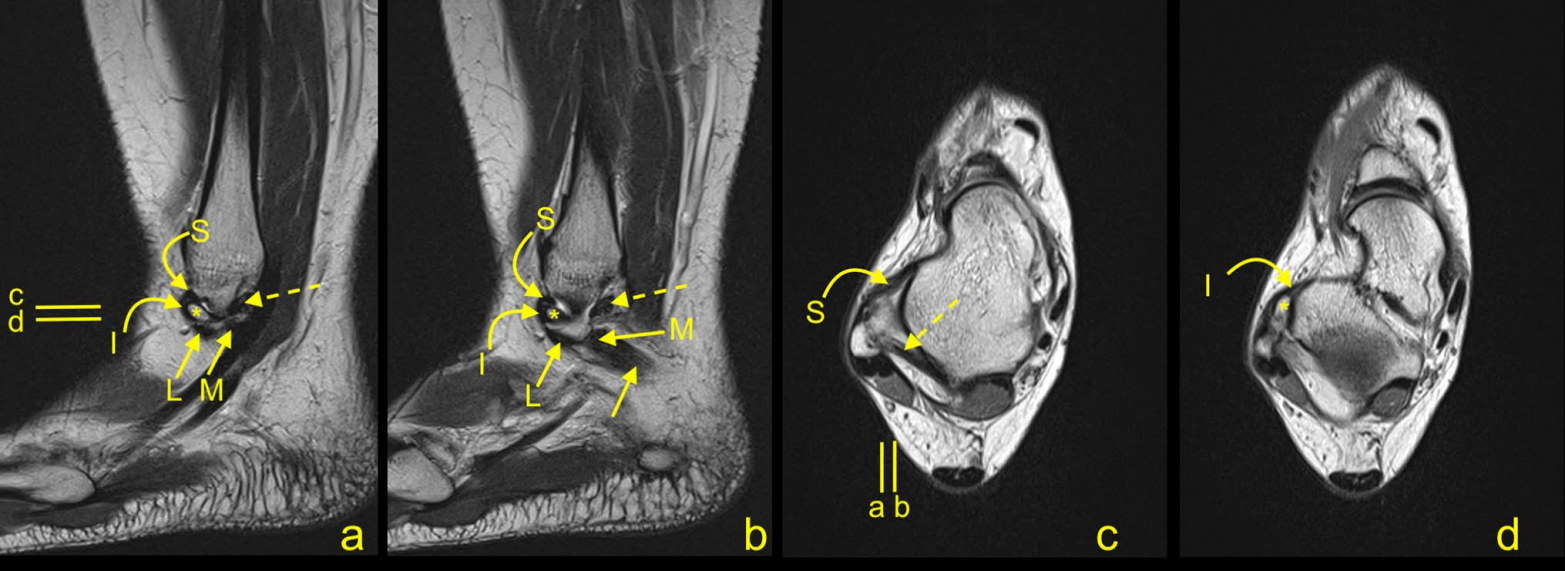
A 20-yeard-old patient with suspicion of ganglion originating from the talonavicular joint, which was not confirmed on the MRI. The presence of the os subfibulare does not change the anatomical relations of the ligaments. ATFL (curved arrow) and CFL (straight arrow). The M-CFL (M) runs anterior to communicate with the ATFL, while the L-CFL (L) attaches to the fibula.
